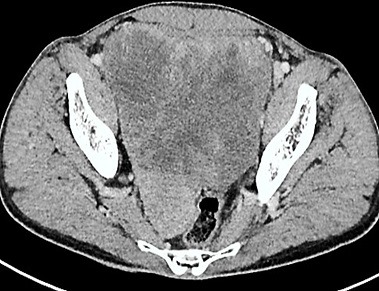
Abdominal CT scan showing a large pelvic mass
